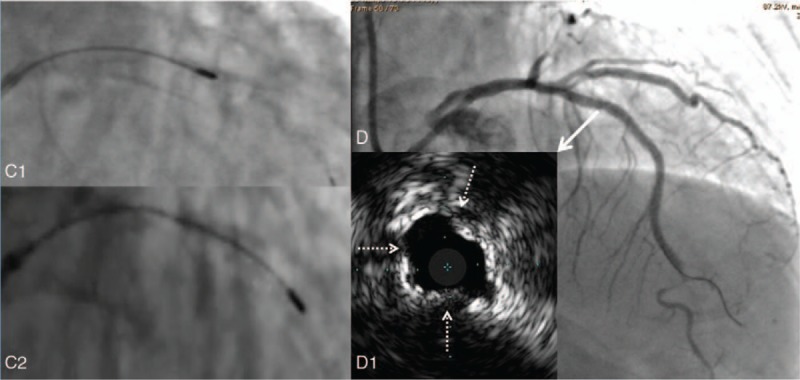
Rotational ablation of the stent C1, rotablator 1.25 mm burr passing through the stented proximal LAD. C2, rotablator 1.5 mm burr passed through the stented proximal LAD. D, after ablation and full expansion with a noncomplaint balloon, the angiography showed an acceptable result. D1, IVUS image confirmed ablation of stent implanted in proximal LAD (solid white arrow) at 1, 9, and 9 o’clock direction (white dotted arrow), but the minimum lumen area was only 5.6 mm^2^. LAD = left anterior descending artery, IUVS = intravascular ultrasound.
